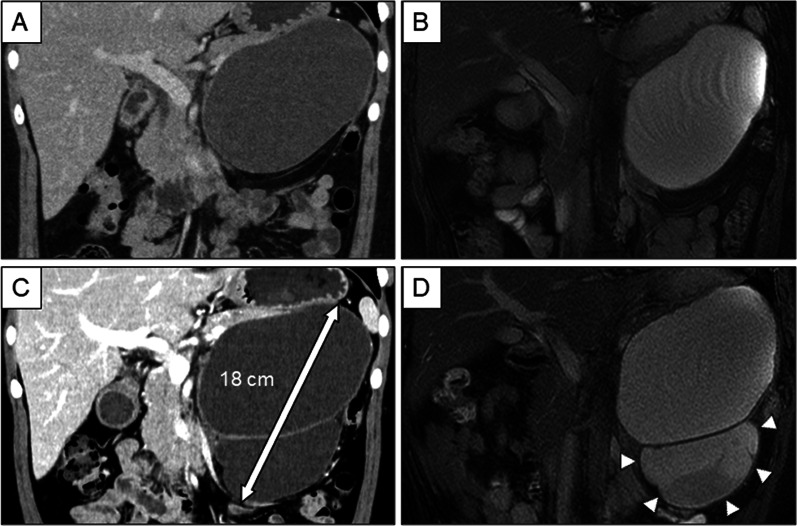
Imaging of pancreas tumor. A Contrast-enhanced computed tomography revealed a 14-cm, well-demarcated cyst. B Magnetic resonance imaging was remarkable for a smooth mass with homogeneous content at the pancreatic tail. C The mass had enlarged to 18 cm within three years due to a new cyst attached to the caudal side of the first cyst. D The solid component inside the new cyst (arrowhead) showed enhancement on diffusion-weighted imaging
